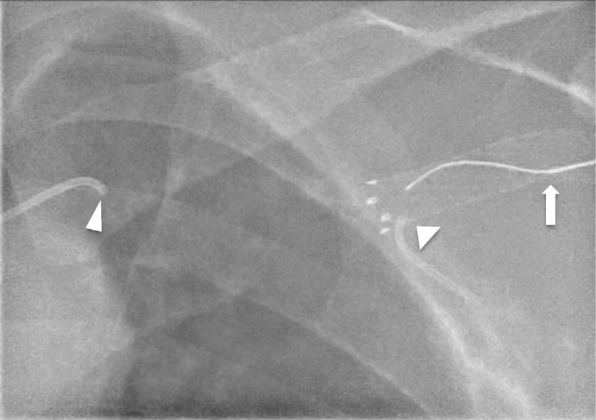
Fluoroscopic image of the occluded left cephalic stent graft, which was accessed with a 21G micropuncture needle under ultrasound guidance. The tip of the needle (arrow) within the occluded stent lumen, with a V18 wire directed centrally. Tips of the antegrade axillary vein (broad arrowhead) and retrograde subclavian vein (narrow arrowhead) 4F Berenstein catheters as shown
